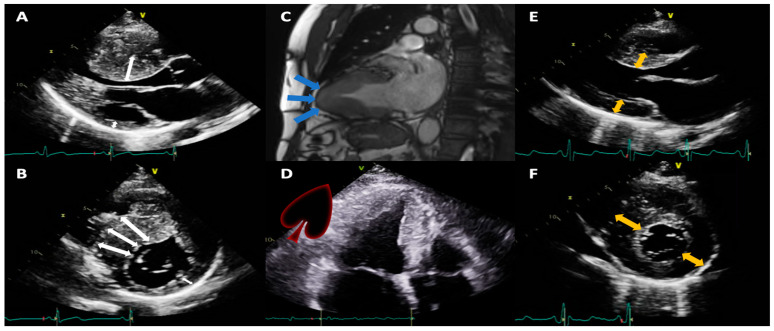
Echocardiographic and CMR images representing various phenotypic expressions of hypertrophic cardiomyopathy (A,B) from a patient with septal asymmetric hypertrophy (white double-headed arrows); (C) (CMR) and (D) in a patient with an apical HCM, which in systole produces a classic spade-shaped ventricular cavity (blue arrows); (E,F) in a concentric phenotype (yellow double-headed arrows). CMR: magnetic resonance imaging; HCM: hypertrophic cardiomyopathy.
